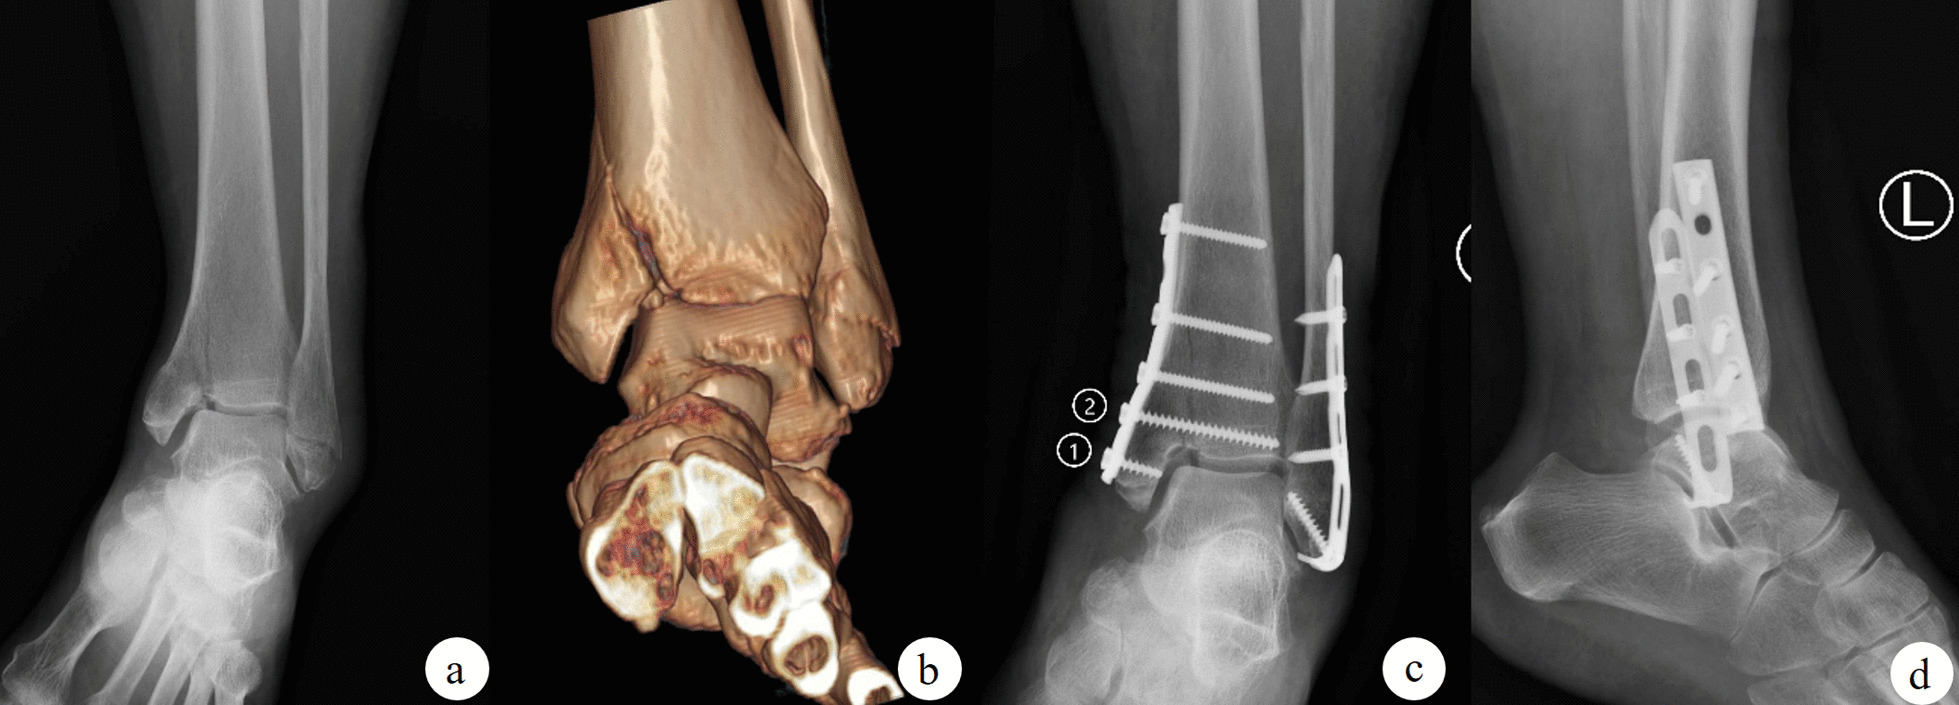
When using the buttress steel plate, the steel plate is prebent into a shape that can be close to the bone surface, and the elasticity and firm structure of the steel plate itself are used to provide fixation, thereby preventing the longitudinal translation of the fracture. However, if only the buttress plate is used for fixation, only one screw c-2 has the function of fixing the fracture because the most distal screw c-1 only fixes the tip of the medial malleolus. a The orthographic X-ray images of the fracture, b the three-dimensional CT reconstruction image after the fracture, and c, d the orthographic X-ray images after buttress plate internal fixation
